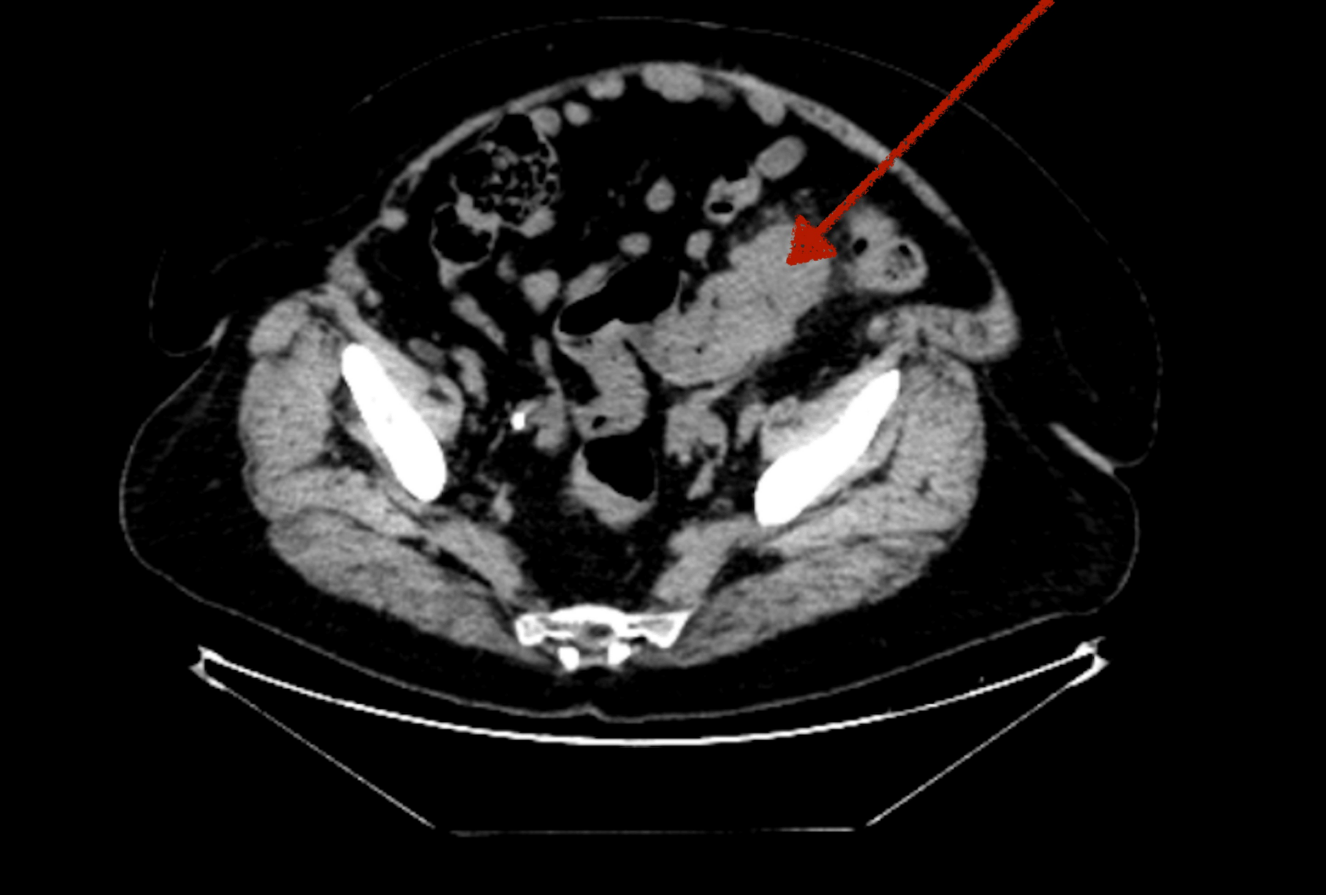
Axial section of the CT chest-abdomen-pelvis, showing the sigmoid mass (red arrow) CT: computed tomography
